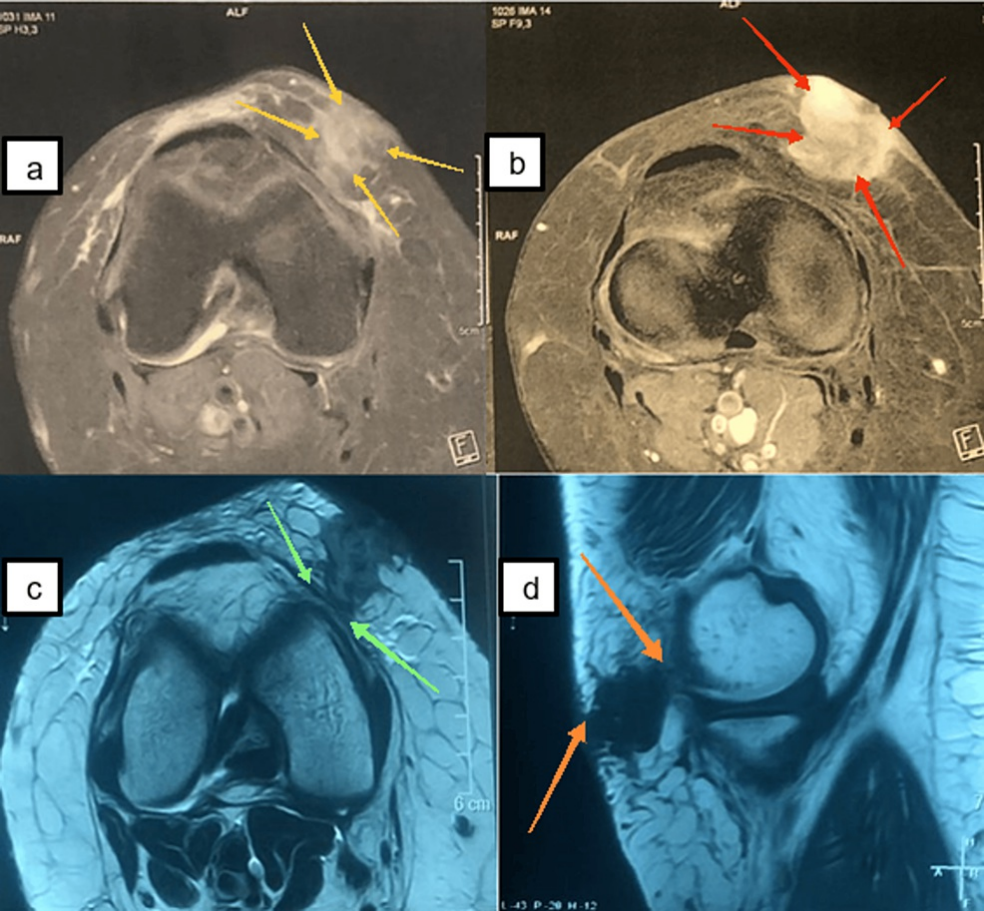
MRI of the right knee shows an oval tissue mass in the medial soft tissues of the right knee opposite the patella (yellow arrows) with deep and superficial development (orange arrows), containing no hemorrhagic components with heterogeneous enhancement after injection of gadolinium (red arrows) and no osteoligamentous, meniscal, or tendon abnormalities in the knee (green arrows). MRI, magnetic resonance imaging
